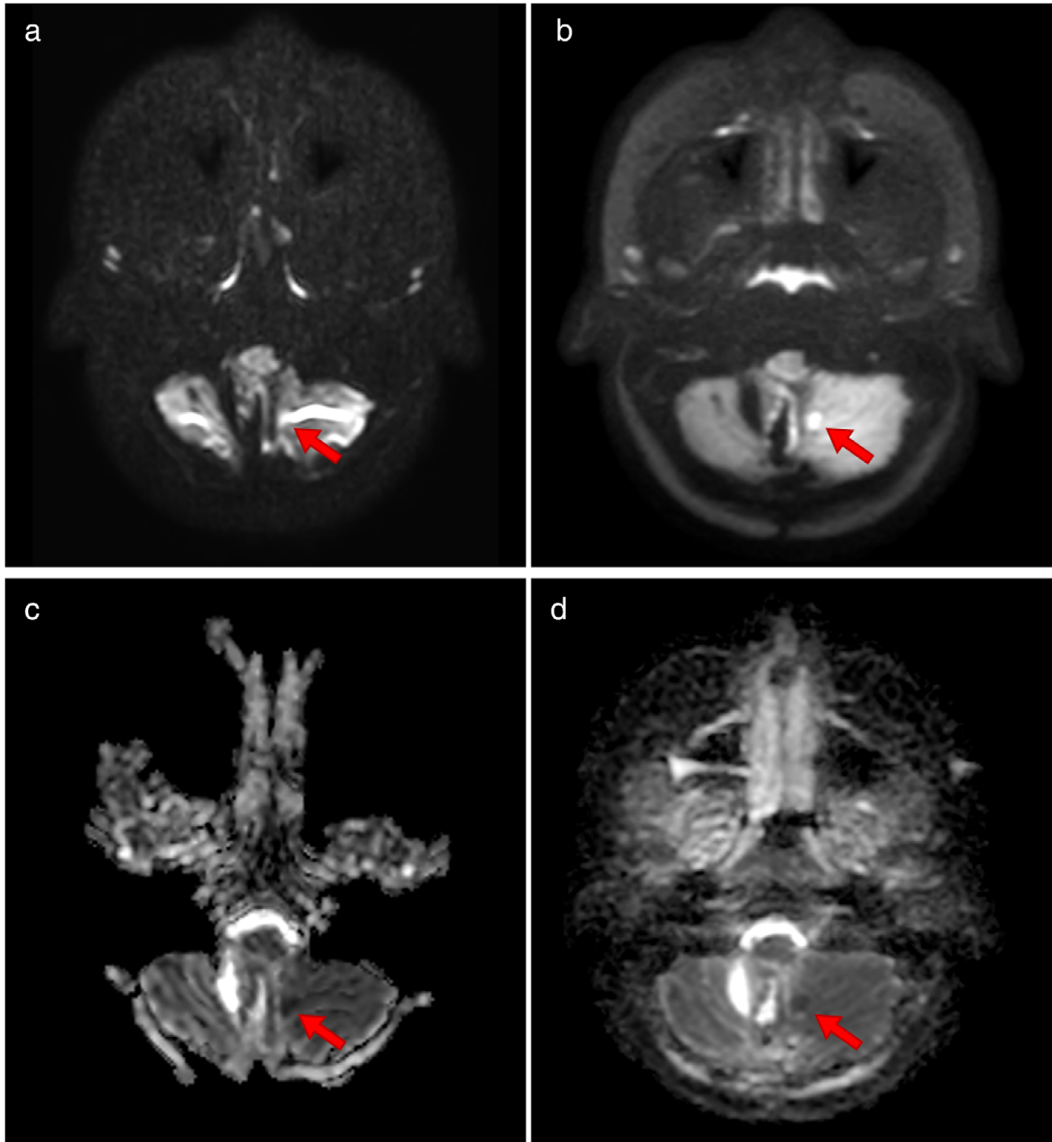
b = 1000 (a, b) and ADC (c, d) images for EPI‐DWI (a, c) and TSE‐DWI (b, d) featuring cerebellar infarct indicated by red arrow.
